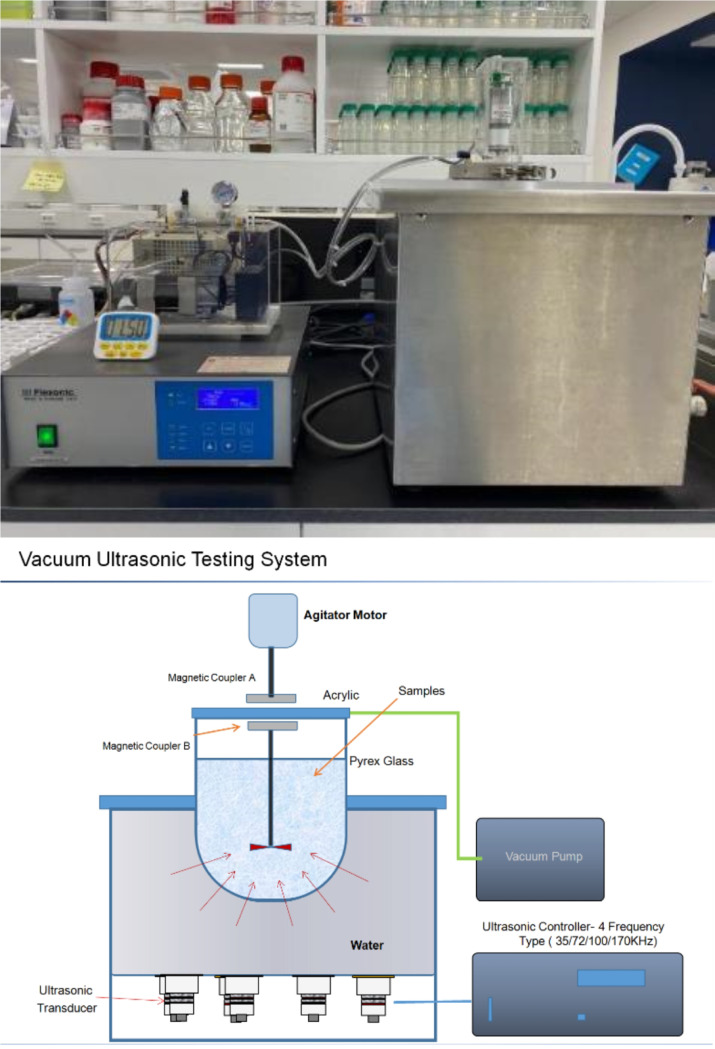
Vacuum Assisted Ultrasonic Stirrer (VAUS^™^, Mirae Ultrasonic Tech., Korea)
